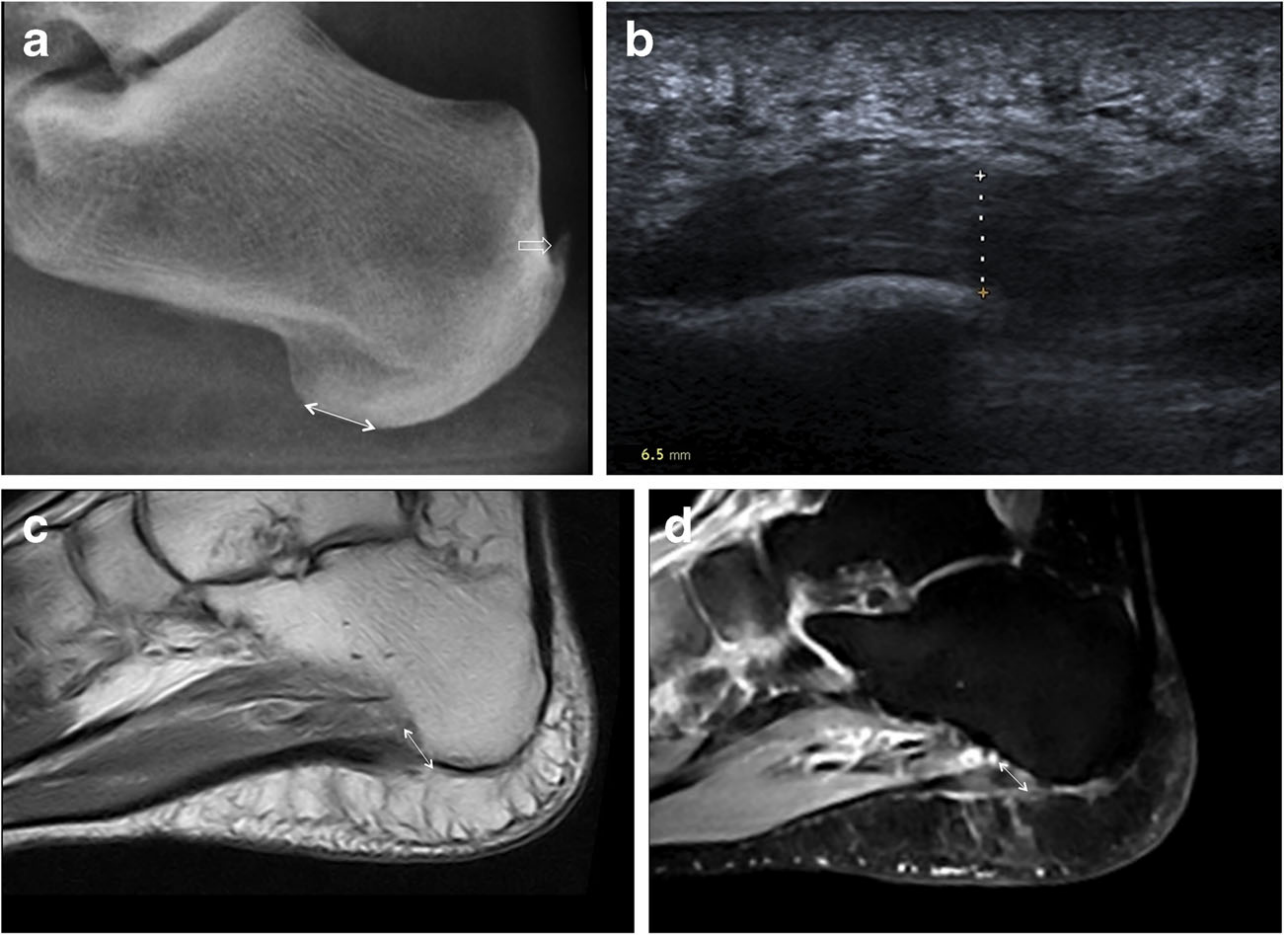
Plantar fasciitis. Lateral plain radiograph highlights an increase in the distance between subcutaneous fat and intrinsic muscles of the foot at the calcaneal insertion of the PF as an indirect sign of plantar fasciitis (*double-head arrow*); calcific enthesopathy of the Achilles tendon is also seen (*open arrow*) (a). On ultrasound, plantar fasciitis presents with PF thickening (*dashed line*, 6.5 mm), a hypoechoic appearance and loss of fibrillar pattern (b). MRI confirms thickening of the PF at its calcaneal origin (*double-head arrow*) with intrasubstance areas of intermediate and high signal intensity on T1-weighted (c) and fluid-sensitive (d) images, respectively
